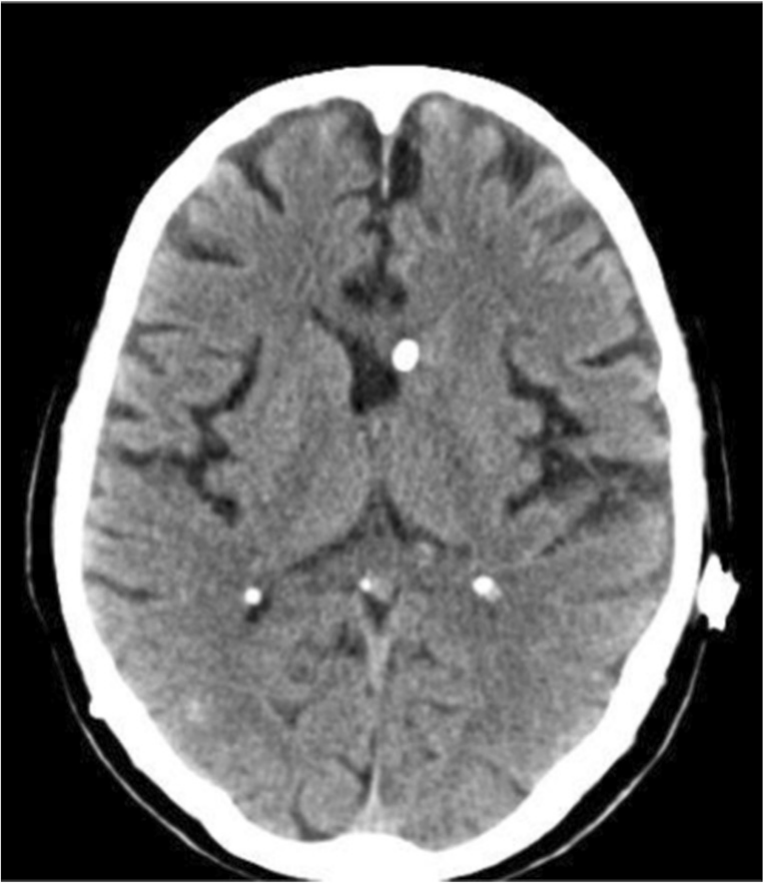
Brain computed tomography scan showing improved ventricular dilatation after ventriculoperitoneal shunting.
